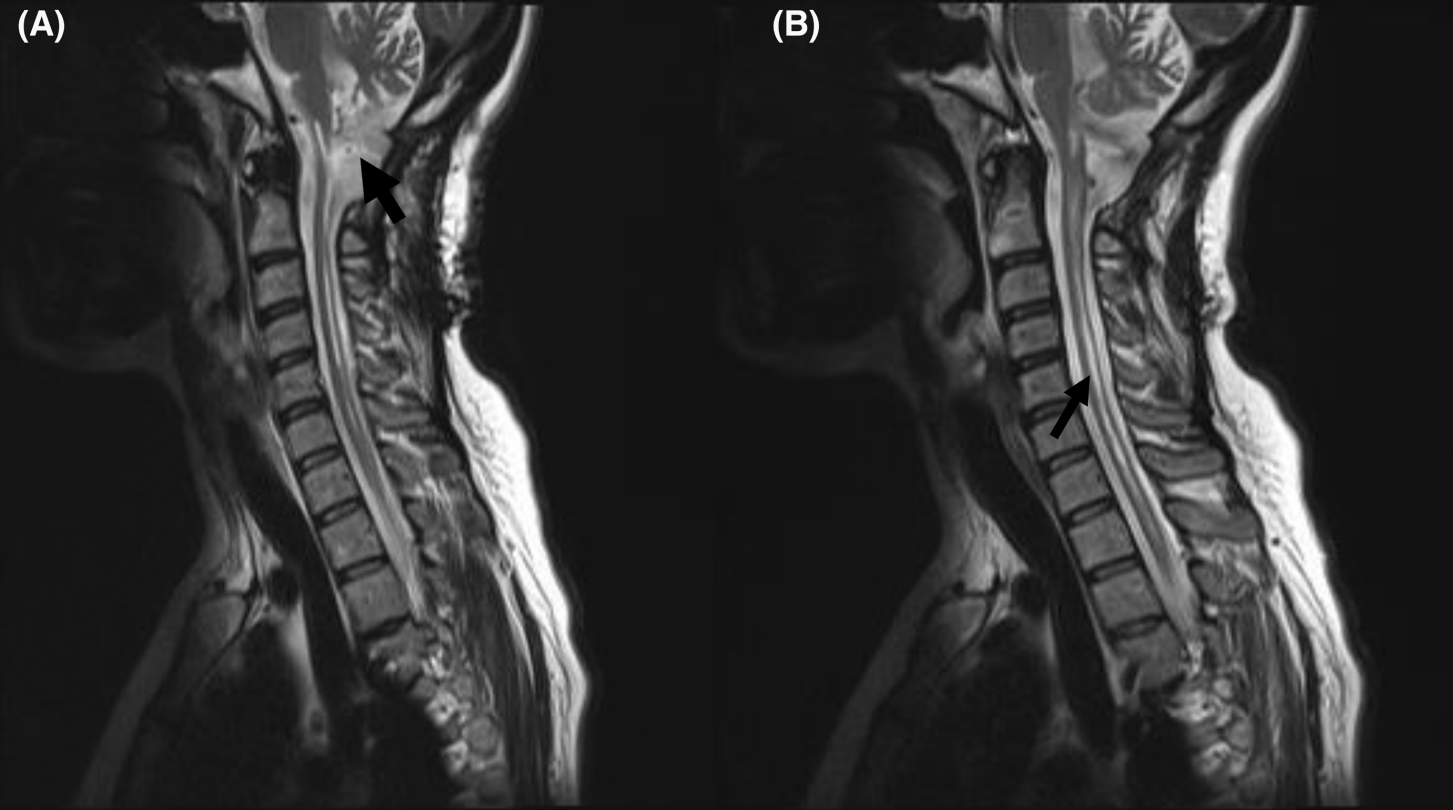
Sagittal T2‐weighted MRI of the cervicothoracic spine demonstrating suboccipital craniotomy and removal of the C1 arc with free CSF flow in the CVJ (arrow) (A); sagittal T2‐wighted MR images of the cervicothoracic juction demonstrating decreased size and diameter of the holocord syrinx when compared to preoperative images (arrow) along with normal CSF flow (B).
